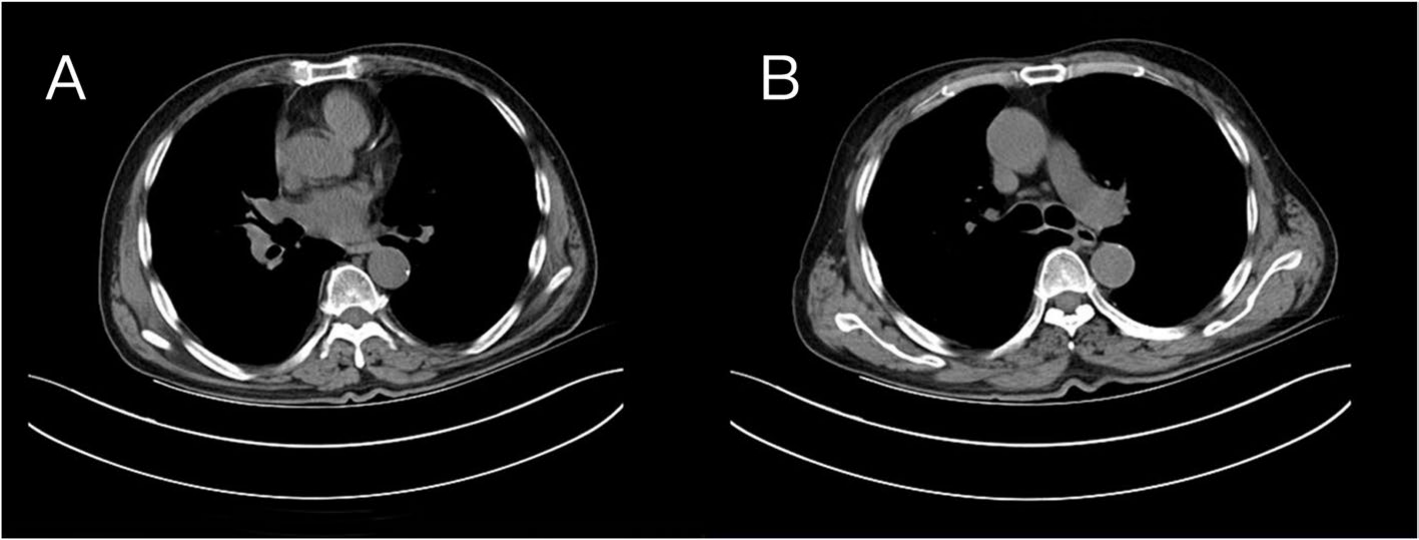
The chest CT imaging after tislelizumab therapy in August 2021.In comparison to that of April 2021, the volume of parabronchial soft tissue masses in the right lung's middle lobe was significantly reduced (A), and enlarged lymph nodes beneath the tracheal carina was significantly reduced (B)
